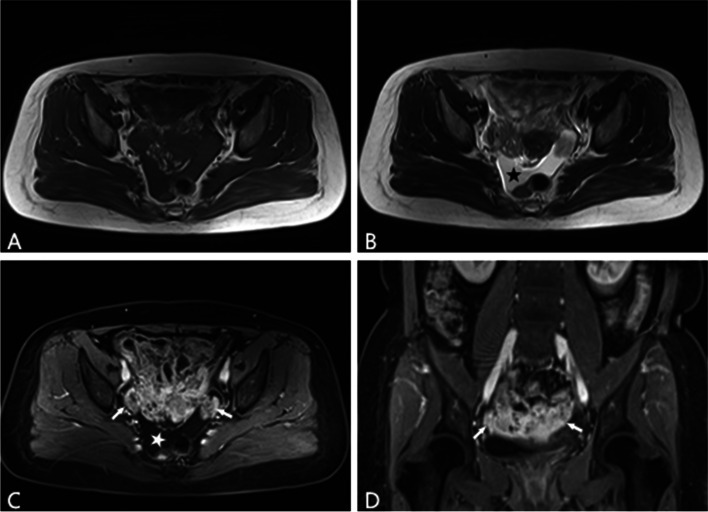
A 52-year-old woman with bilateral high grade of serous ovarian carcinoma. A–B Irregular solid mass on the bilateral ovarian regions with unclear boundaries present isointensity and slight hyperintensity signals on axial T1WI and T2WI, respectively. Ascite in rectum lacuna (pentastar) was found. C–D Axial and coronary contrast-enhanced fat-suppressed T1-weighted MR image shows markedly and unevenly enhanced solid component within complex solid and follicular mass in pelvis (arrows)
